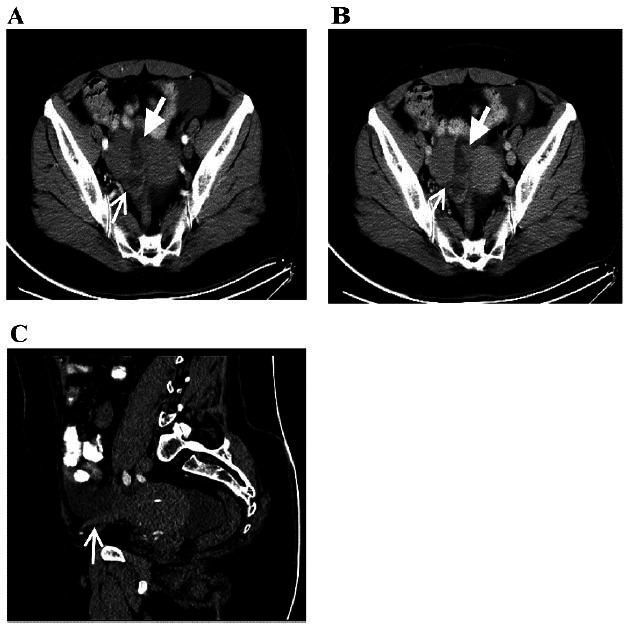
Primary fallopian tube carcinoma in a 54-year-old woman. Computed tomography images (A–C).
